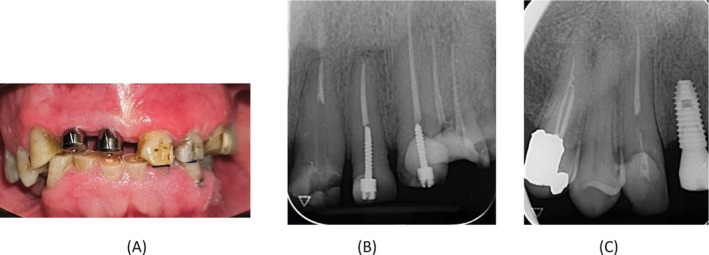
(A) Preoperative clinical photograph. (B) Perioperative periapical radiograph of tooth #9 showing a long fiber post extending into the apical third of the canal. (C) Perioperative periapical radiograph of tooth #6 showing a short root canal filling.
